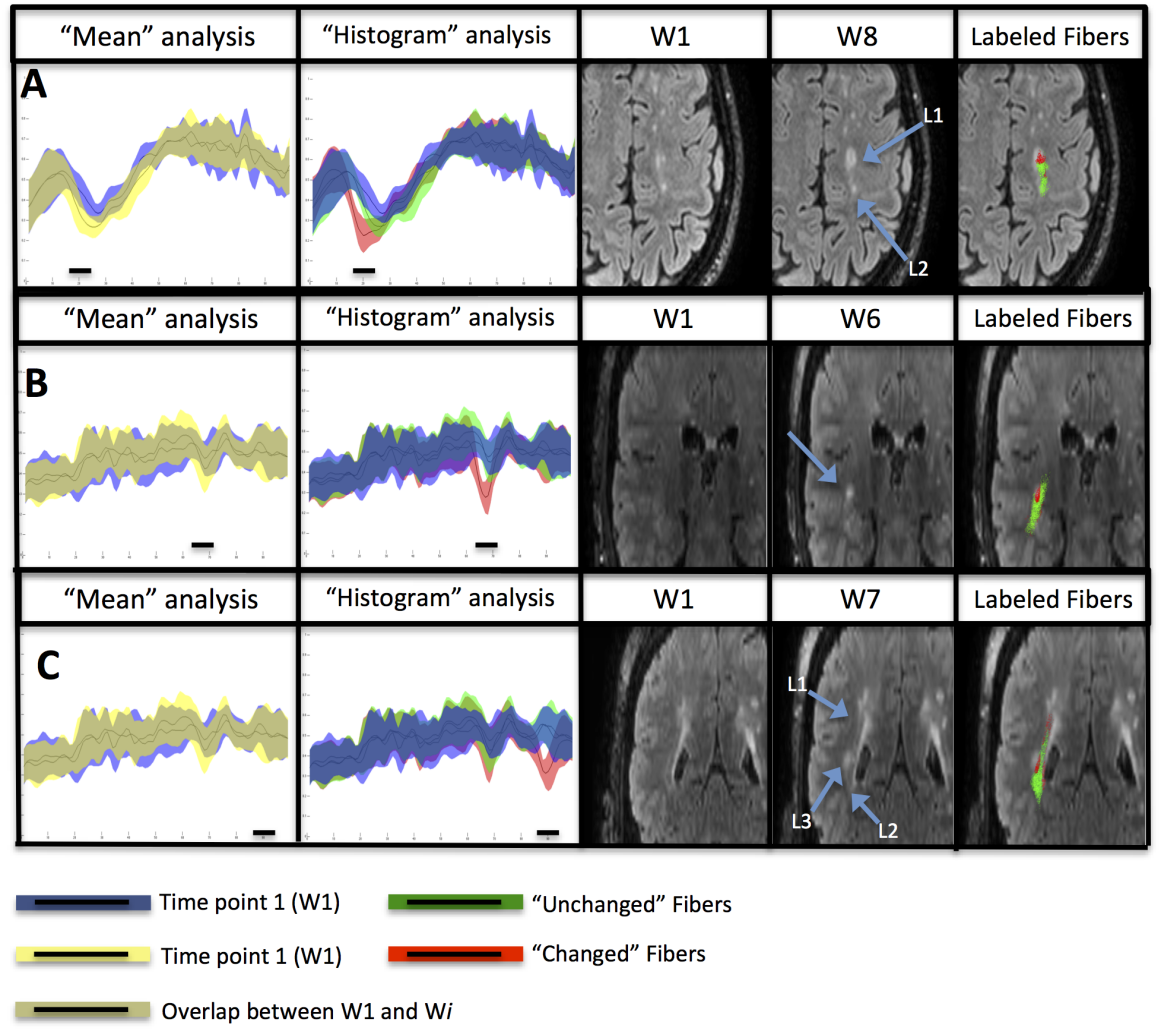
Detection of longitudinal variations by applying the “mean” and “histogram” methods. (A) On the left CST of Patient1 between W1 and W8 time-points, detecting a change in two preexisting lesions (L1, L2); (B) On the right IFOF of Patient2 between W1 and W6 detecting a new lesion; (C) On the right IFOF of Patient2 between W1 and W7 detecting a change in two preexisting lesions (L1, L2) and the apparition of a new lesion (L3). Lesions are shown on FLAIR images. Fiber-subsets labeled as “unchanged” (green) and “changed” (red) are shown on top of FLAIR images.
